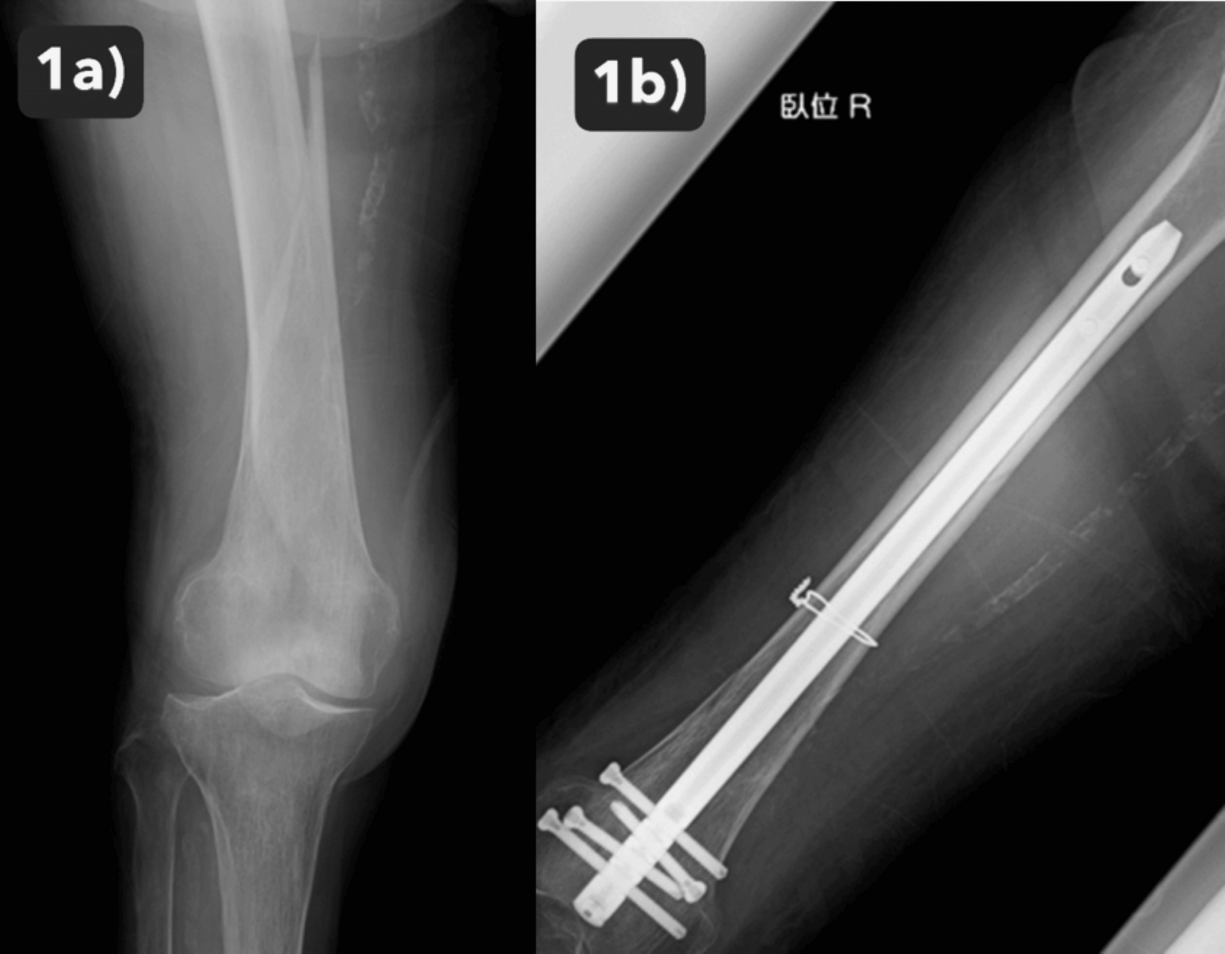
Right femur fracture at initial evaluation and postoperative radiograph of the right femur 1a) Right femur fracture at initial evaluation; anteroposterior and lateral radiographs showed the displaced right femoral shaft fracture. 1b) Postoperative radiograph of right femur; The fracture was fixed with a retrograde intramedullary nail with cerclage wiring.
